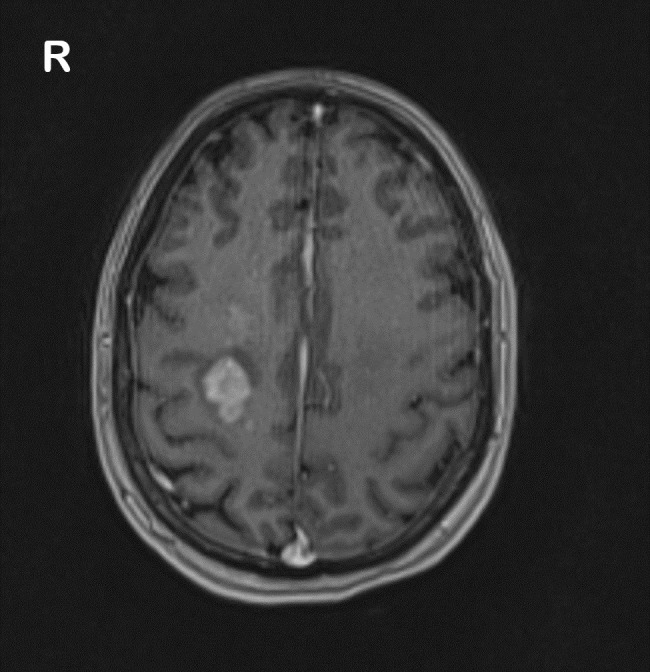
MRI of the brain demonstrating a right-sided mass involving the basal ganglia and thalamus.
